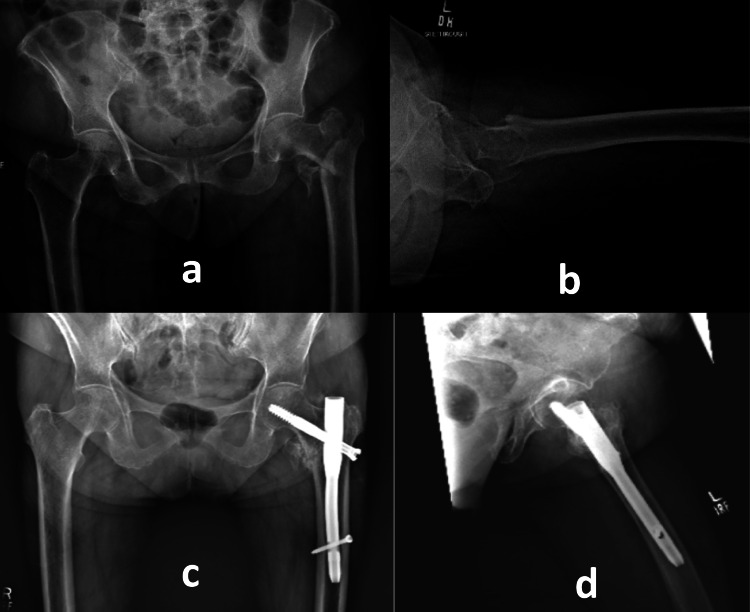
Pre-operative radiographs (AP view and lateral view) of a 75-year-old patient showing unstable per-trochanteric fracture of left femur (a & b) and Post-operative radiographs (AP view and lateral view) at 6 months showing Intertrochanteric fracture of left femur fixed via CRIF with Intramedullary nail showing radiological signs of healing with RUSH score of 20 and HHS of 80 (c&d).
